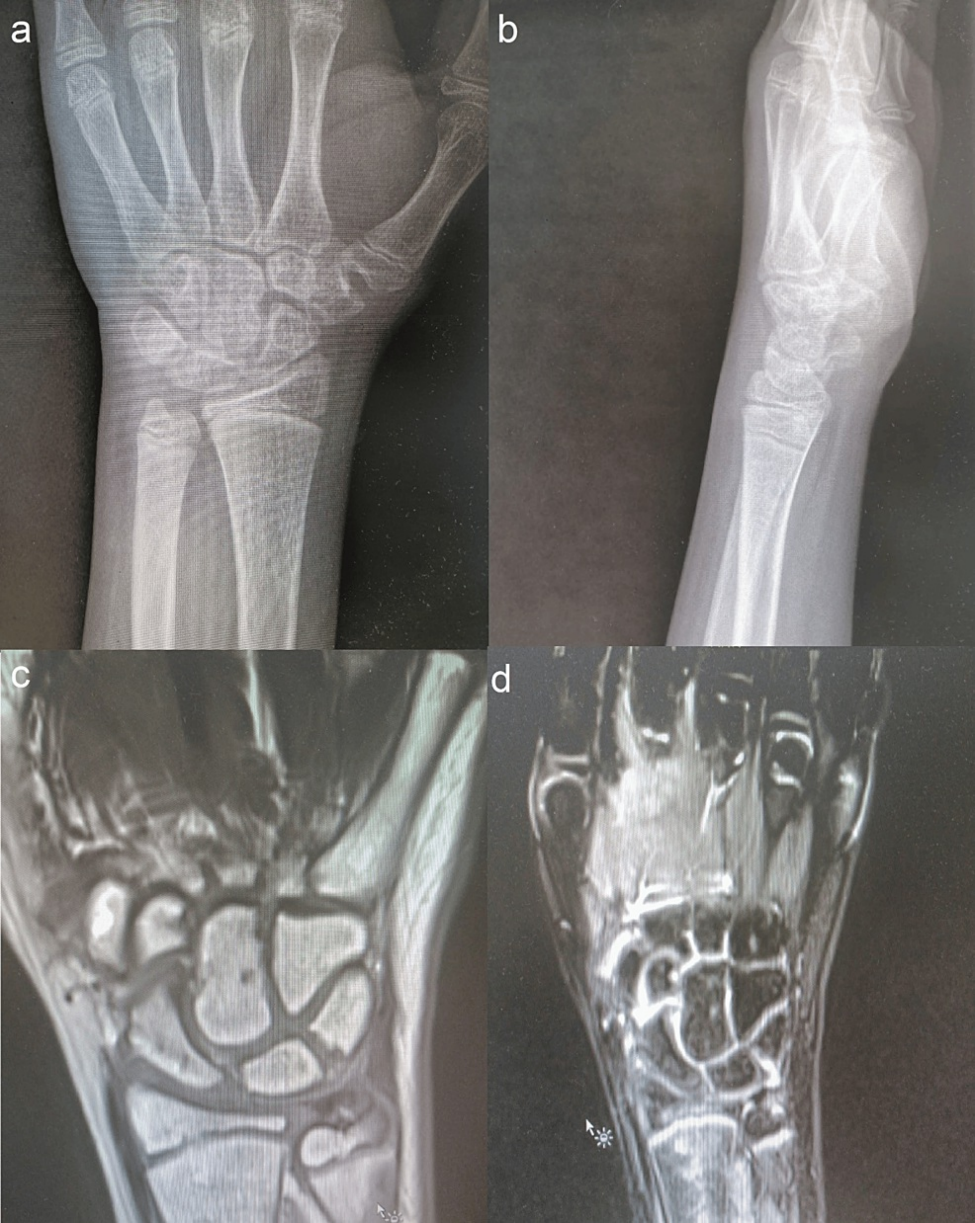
(a, b) Posterior-anterior and lateral left wrist postoperative radiographs at six months showed restoration of bone density. (c, d) Coronal T1 and T2 left wrist MRI showed signs of revascularization, including the return to normal intensity.
